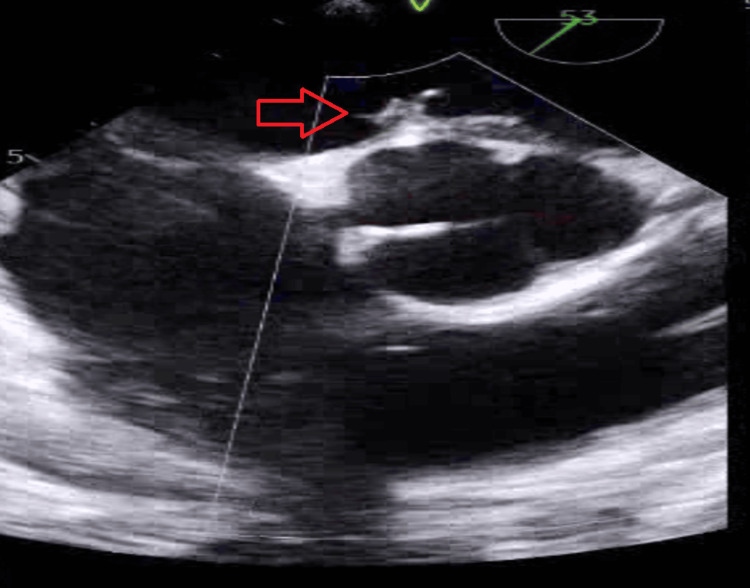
Initial transesophageal echocardiography (TEE) image from the hospitalization. The arrow demonstrates a second infective vegetation on the left atrial wall extending into the aortic valve annulus on TEE.
